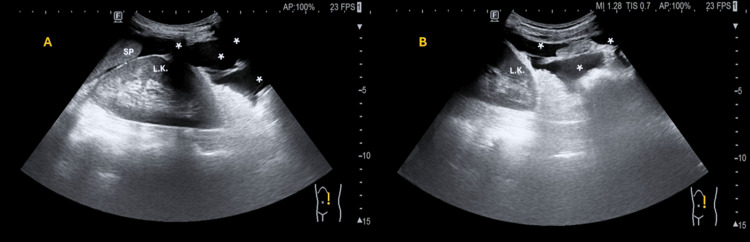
Abdominopelvic ultrasound on a normal left kidney in two longitudinal planes: panels A and B. Sagittal views of the left kidney in panels A and B show a reniform organ with preserved corticomedullary differentiation and an intact echogenic renal sinus  without hydronephrosis. It is important to note that hydronephrosis being only unilateral (on the right) indicates that the mass is not intravesical, that is, the mass is extrinsic to the bladder and slightly to the right sagittal plane. A thin anechoic rim of perinephric free fluid surrounds the lower pole and extends along the lateral margin of the kidney, consistent with free fluid identified by (*). The inferior tip of the spleen (SP) is visualized just superficial to the upper pole of the left kidney and appears sonographically unremarkable.
